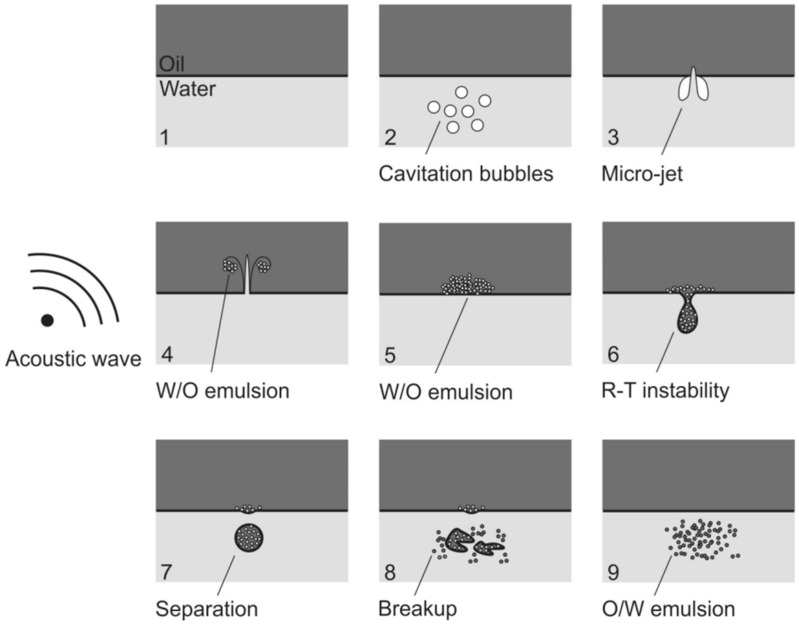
Proposed mechanism for ultrasound-assisted oil-in-water emulsification. (1–5) The collapse of cavitation bubbles causes water droplets to disperse into the oil phase, forming a water-in-oil emulsion. (6–9) Due to Rayleigh–Taylor instability, some of the oil phase disperses into the aqueous phase, and the droplets are broken down to smaller particles through continued sonication. These steps are repeated until a true oil-in-water emulsion is formed. W/O: water-in-oil; R-T: Rayleigh–Taylor; O/W: oil-in-water. Reproduced from the study published by the authors of [48].
